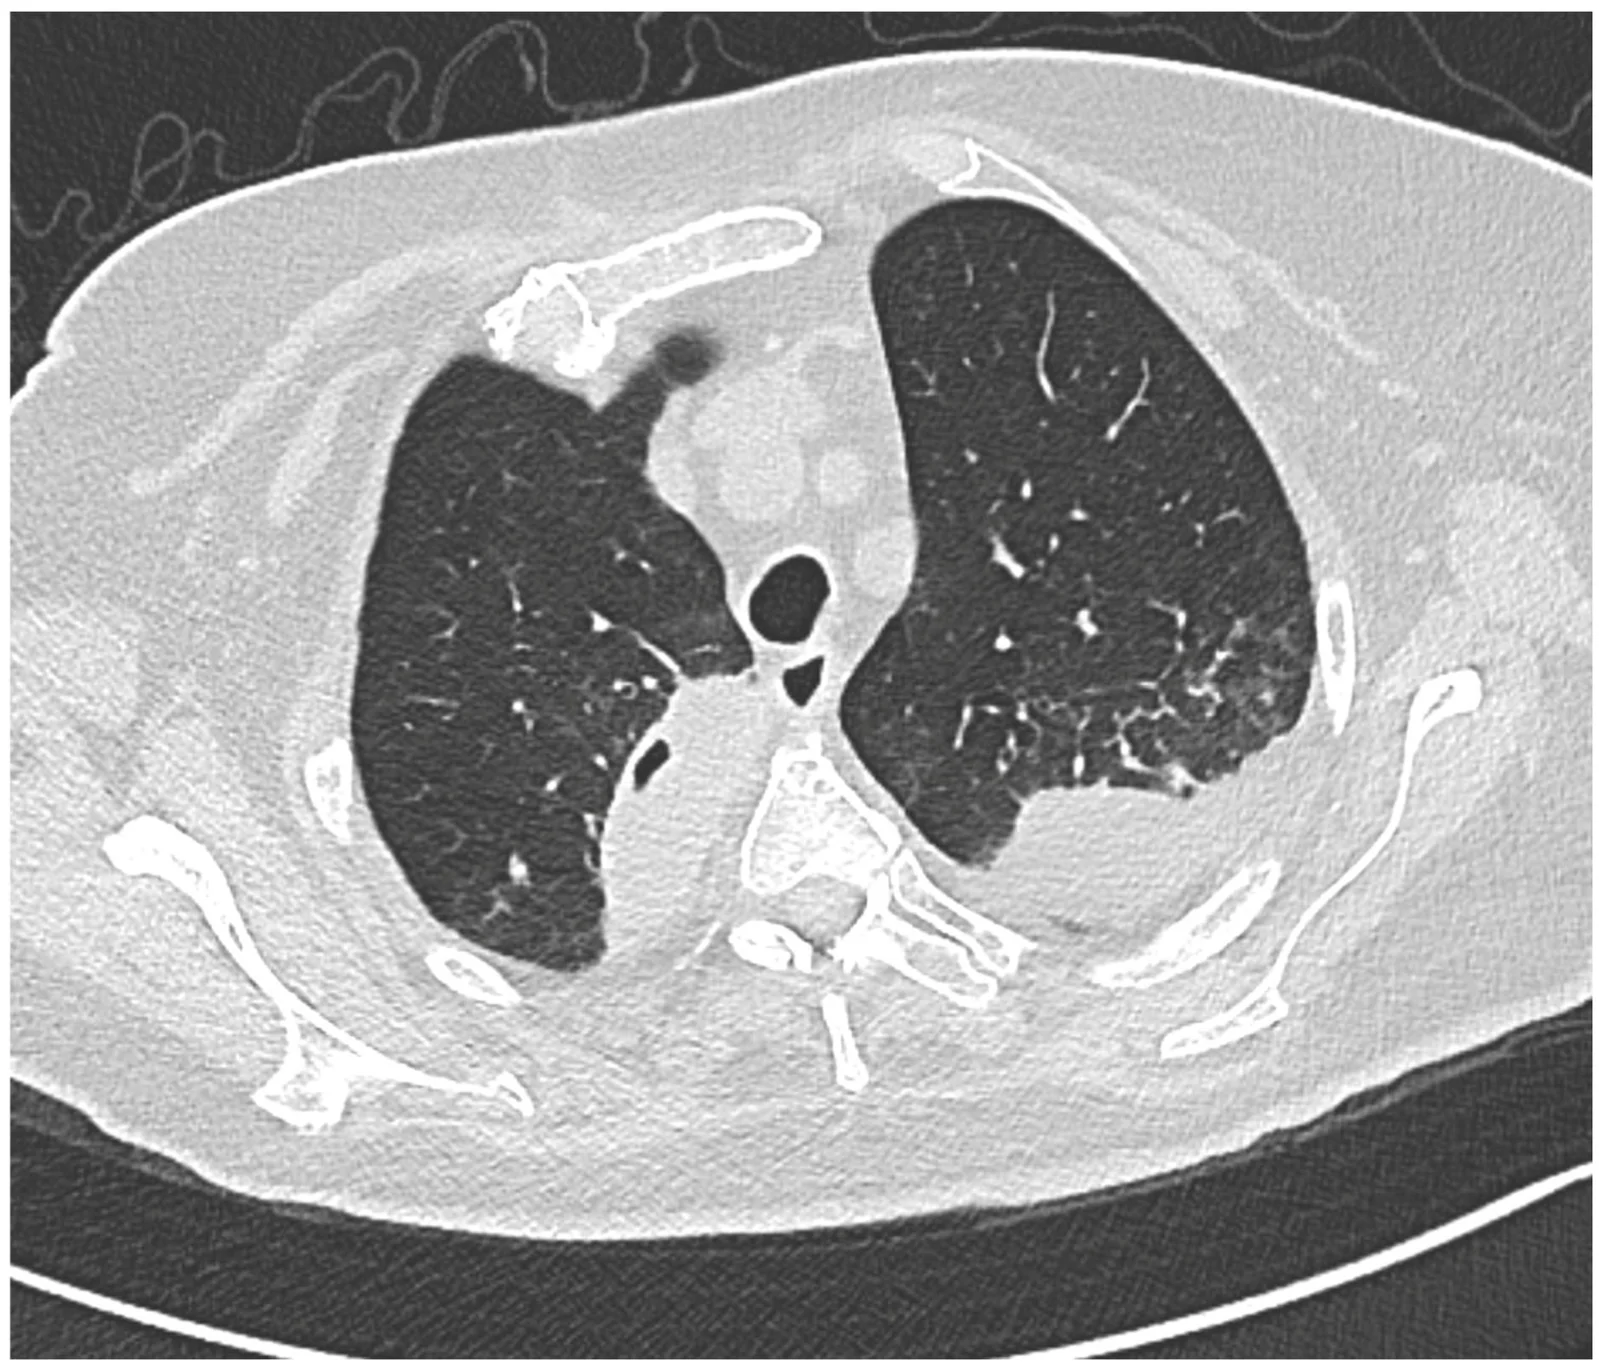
HRCT, axial plane. This patient was affected by bilateral pulmonary *Nocardia* infection. HRCT shows mass-like consolidations in the upper lobes; in the right lung the air crescent sign can be observed while in the left lung are associated interlobular septal thickening and pleural effusion. The air crescent sign makes the radiological differential diagnosis with *Aspergillus* pulmonary infection very difficult.
